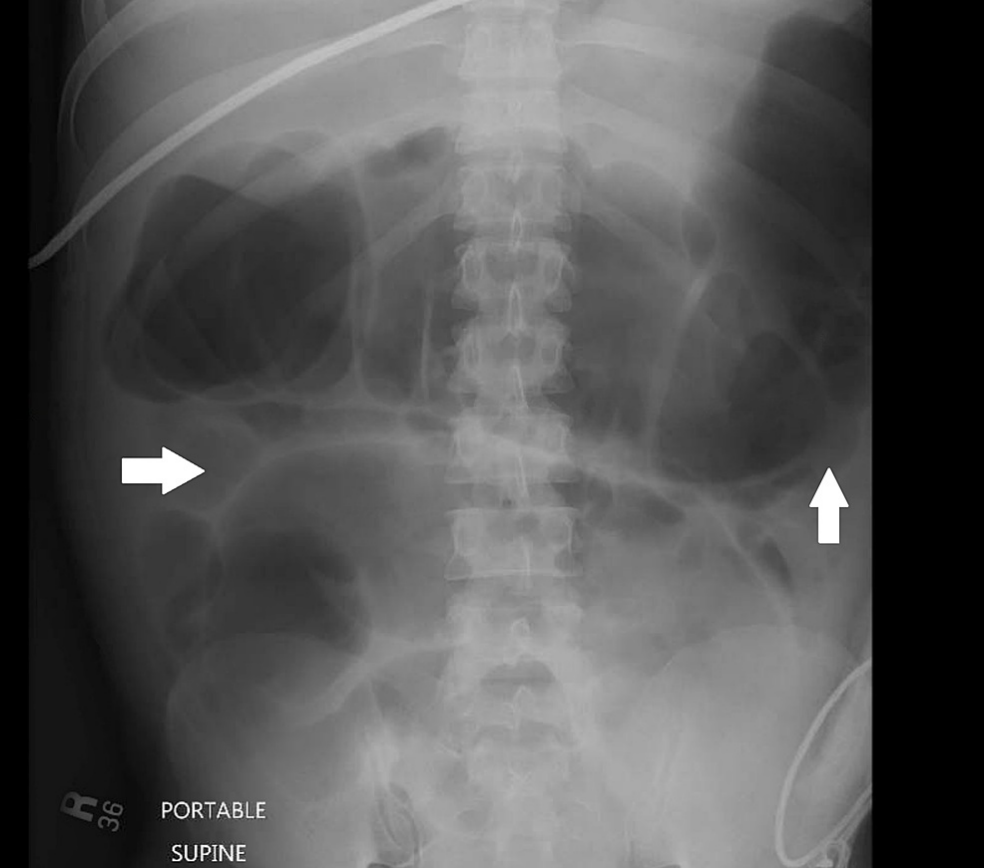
X-ray abdomen showing marked gaseous distension of the stomach and intestinal loops.
